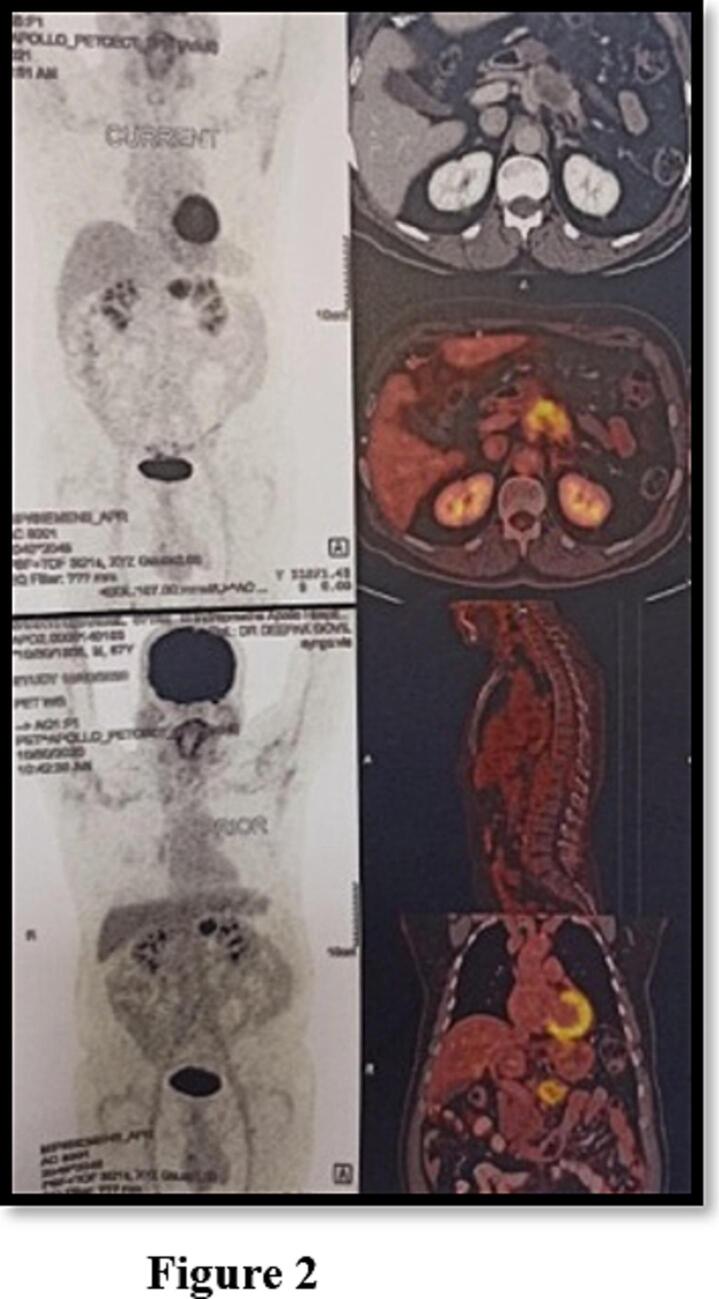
PET-CT scan demonstrating intense FDG uptake in the pancreatic body tumor with no evidence of distant metastases. The hypermetabolic signal correlates with the mass seen on cross-sectional imaging and supports surgical exploration in a non-metastatic setting.
